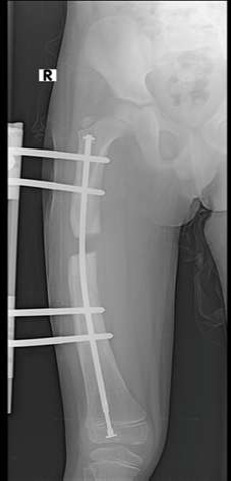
anteroposterior femur X-ray—start of distraction of the femur
